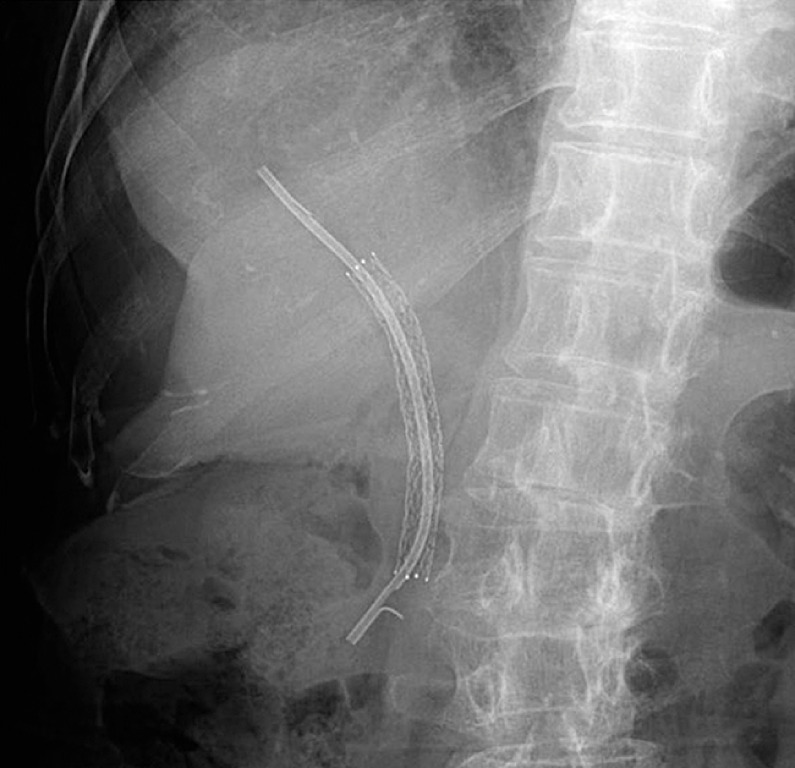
X-ray of 58-year-old woman showing possibly occluded plastic stent coaxial within a metallic stent, placed for distal biliary obstruction caused by malignant lymphoma.
